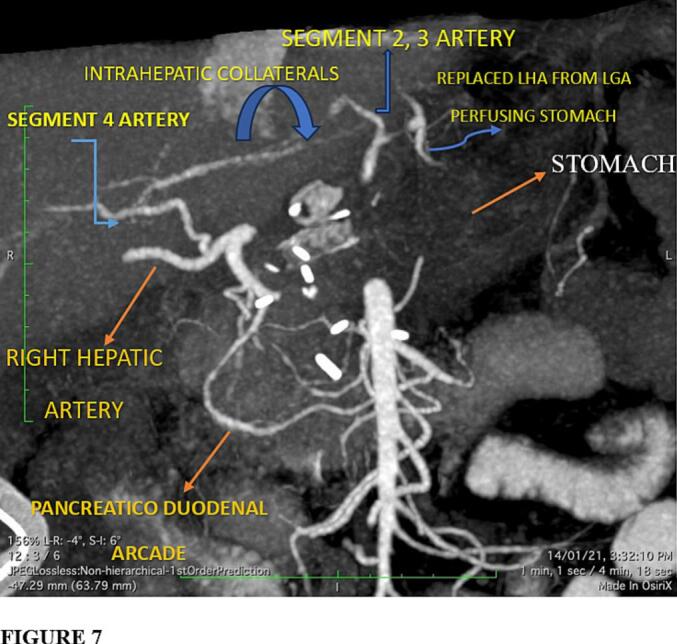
Postoperative contrast-enhanced CT on day 7 demonstrating preserved gastric and hepatic arterial perfusion. No ischemic changes were identified, validating the intraoperative perfusion assessment.
